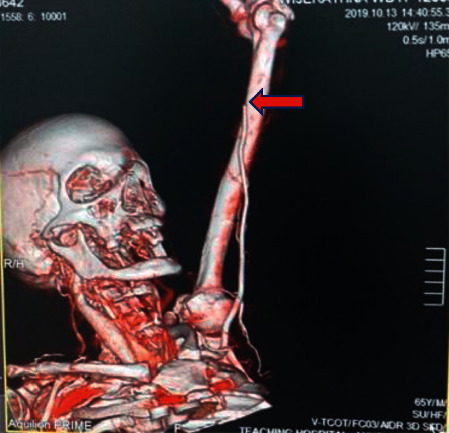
CT angiogram showing the left brachial artery occlusion (red arrow).
